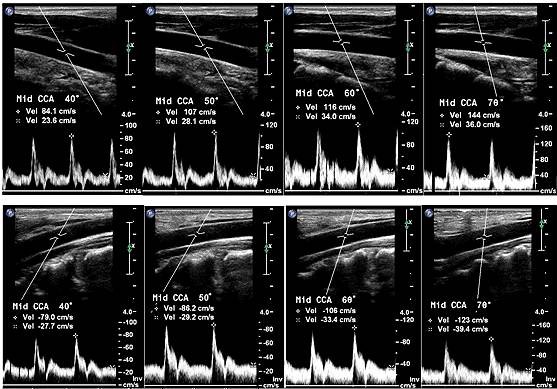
Effect of Doppler Examination Angle. All 8 images were acquired from the same location in the same artery within the same examination period using the Doppler equation to correct for the geometric angle between the ultrasound beam and the artery axis. All duplex Doppler ultrasound instruments use this method to adjust the velocity measurement value reported. This systematic measurement bias (higher values for larger angles) appears in all peripheral arteries. The sole exception is measurements distal to long straight arteries such as the distal superficial femoral artery. For equal Doppler angles, steering the ultrasound beam toward the feet (upper row) appears to provide similar values to steering the ultrasound beam toward the head (lower row), if the same Doppler angle is used, but the effect of this variable should be quantified.
